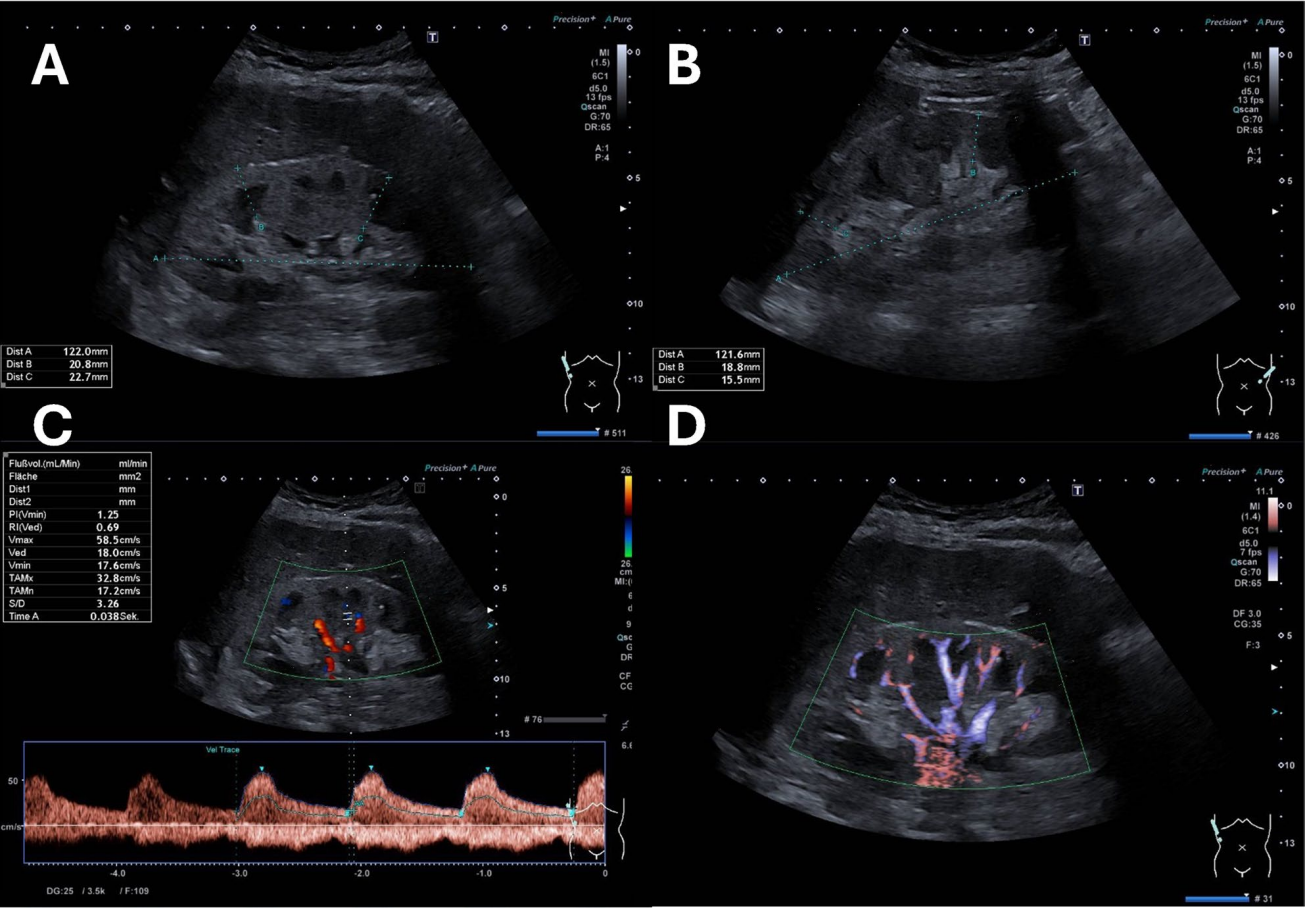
Duplex sonography at the time of admission showing enlarged and swollen kidneys with normal blood flow
